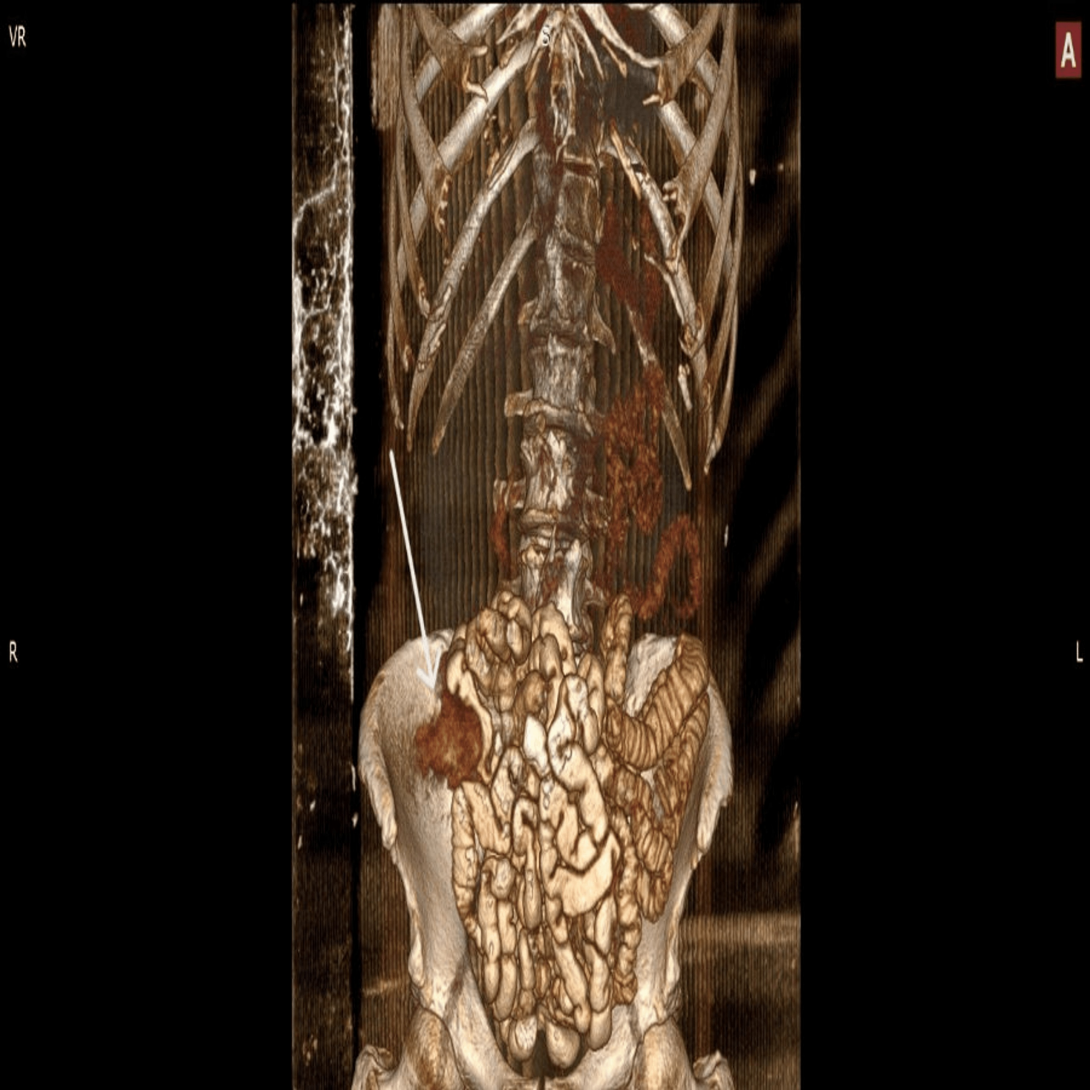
Abdominal CT scan 3D reconstruction showing the lesion CT: computed tomography
